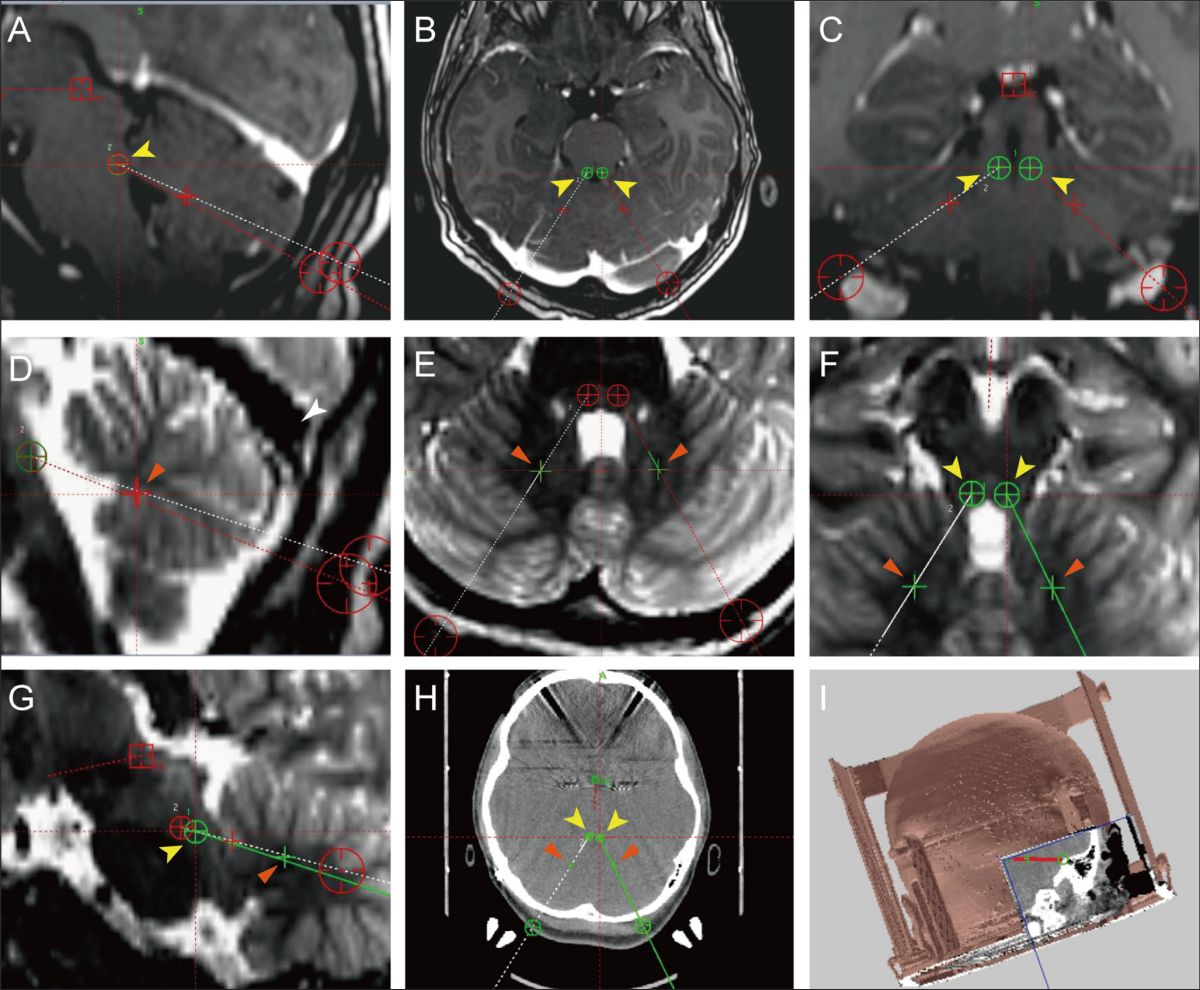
Determining the coordinates of the target superior cerebellar peduncles (SCPs) and dentate nuclei (DNs). The yellow arrows are directed to the SCPs, and orange arrows are directed to the DNs. The stereotactic coordinates of the SCP were identified on the sagittal section as the end point of the SCP to the brainstem (z) (2A) in line with the floor of the fourth ventricle on the horizonal section (y) (2B) and 3 mm lateral from the midline of the coronal section (x) (2C). Coordinates of the DN region were directly under the fastigium of the fourth ventricle on the mid-sagittal section (z, y) (2D) and 13 mm lateral from the midline on the T2 horizonal image (2E). The trajectory was planned using SCPs as the primary target. The coronal and sagittal angles were adjusted to attain a trajectory that traversed the origin of the SCP and target the DN. The trajectory was identified in the horizonal (2F) and sagittal sections (2G), and the entry point was 1 cm away from the sigmoid sinuses in the MRI image and at least 1 cm away from the posterior fixation posts and posterior frame bar in the CT image (2H). The 3D trajectory is shown in (2I).
